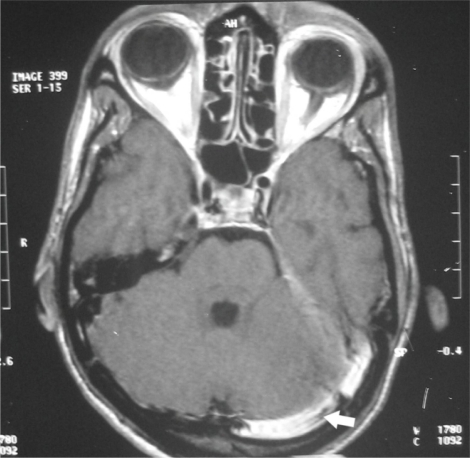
MRI scan showing (arrow) occlusion and dilatation of left transverse and lateral sinus (with permission from Indian journal of Otolaryngology & head and neck surgery).
